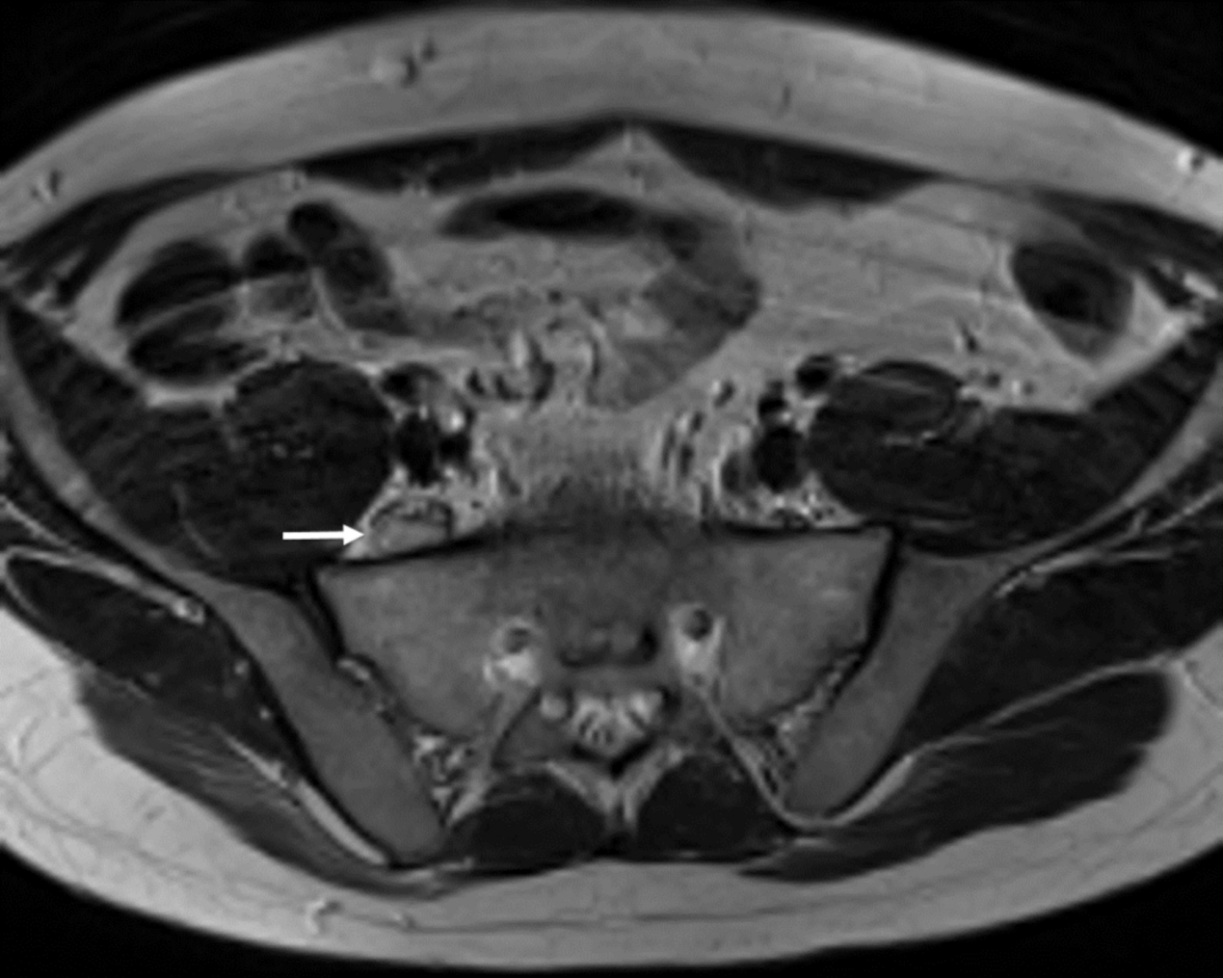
Right pre-sacral mass Axial T2 weighted magnetic resonance imaging (MRI). Retroperitoneal right pre-sacral mass (white arrow) measuring 18 x 8 x 30 mm and displacing the nerve fibres anteromedially suggesting a nerve sheath tumour
